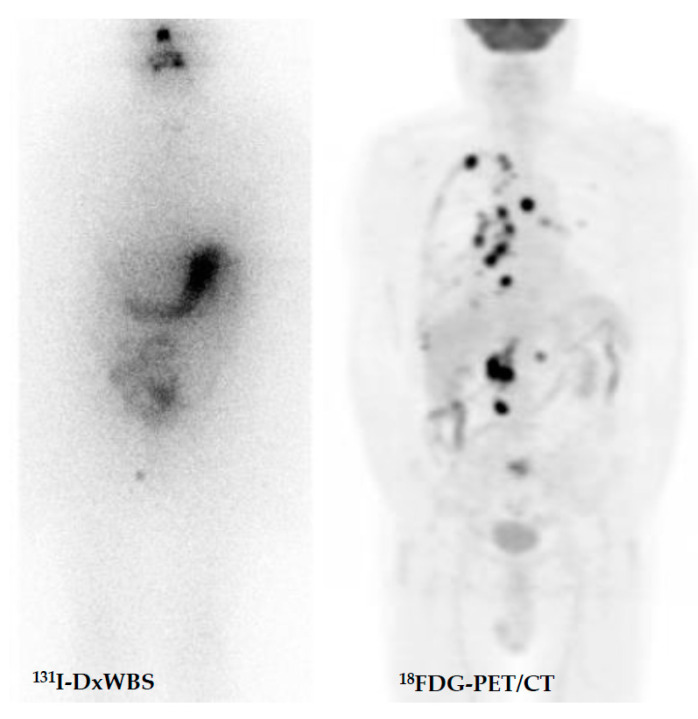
DTC patient with increasing thyroglobulin and negative diagnostic whole-body scan after thyroidectomy and radioiodine therapy. [^18^F]FDG-avid relapsing disease.
